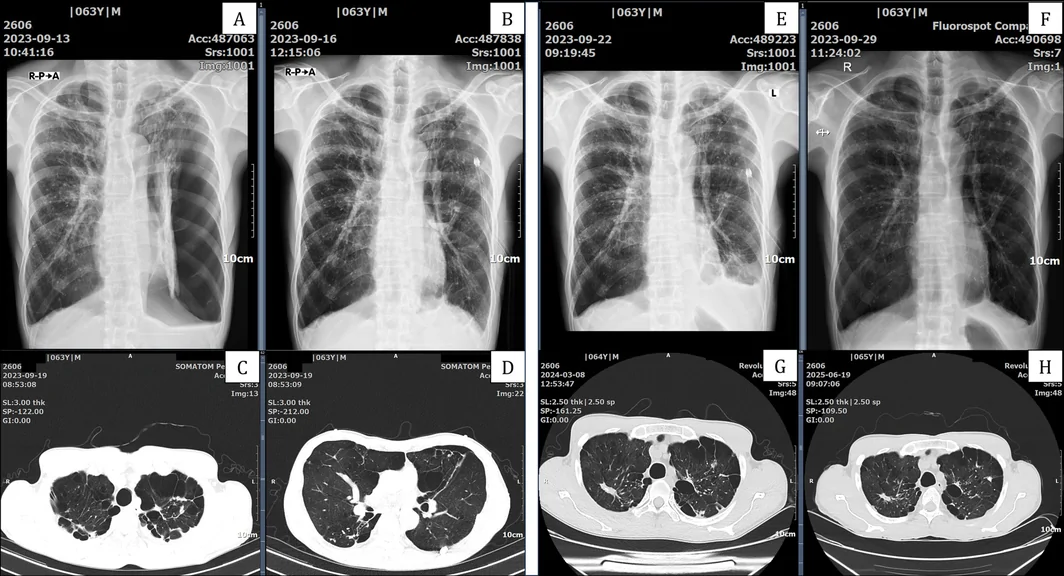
Serial chest imaging studies: (A) chest radiograph on admission; (B) 3 days after ICD insertion; (C–D) chest HRCT showing extensive bilateral bullous emphysema; (E) 2 days after pleurodesis; (F) 1 week post‐pleurodesis; (G) chest HRCT 6 months after pleurodesis; and (H) chest HRCT 20 months post‐pleurodesis.
